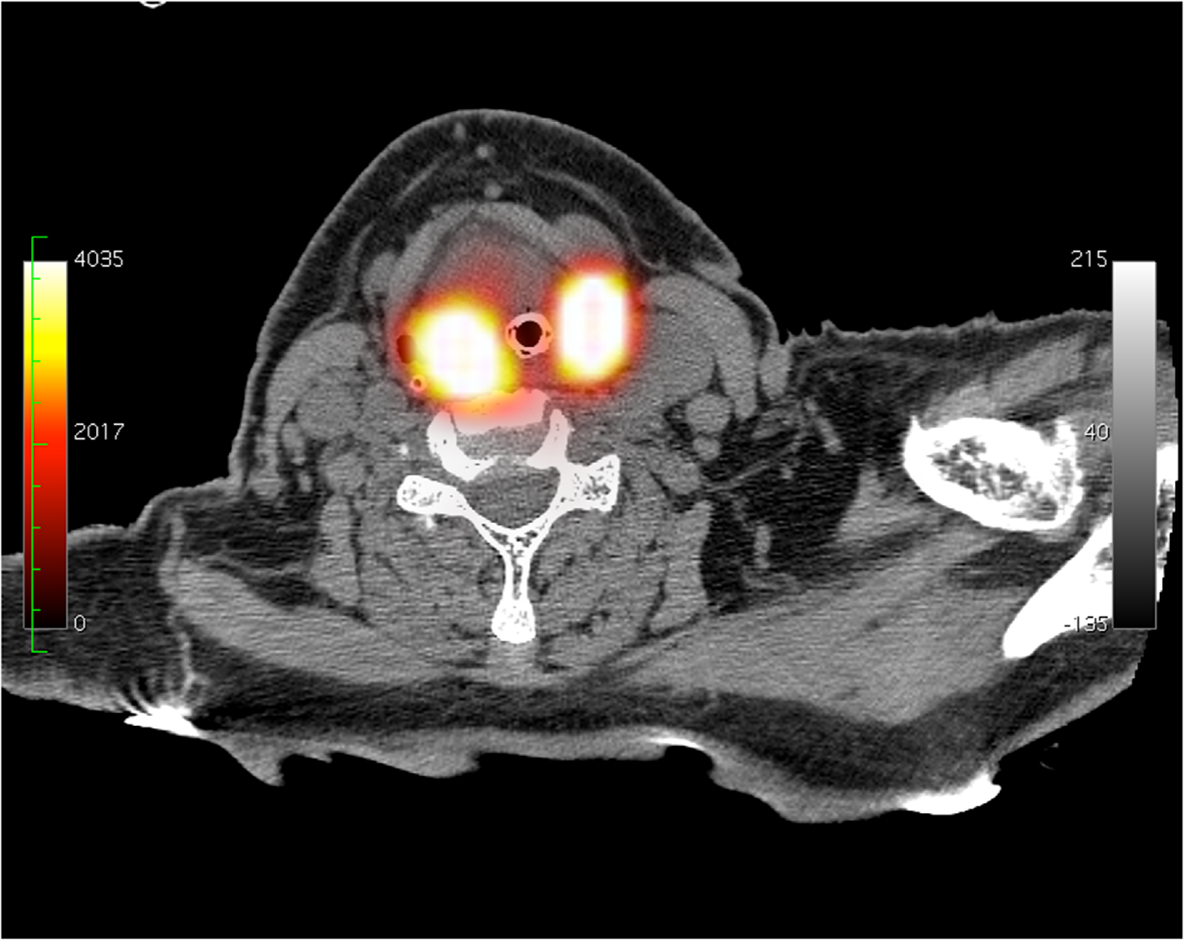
Axial SPECT/CT slice, patient 6. No apparent migration is depicted in level 3 left, probably due to intense activity in neighbouring tumour.
